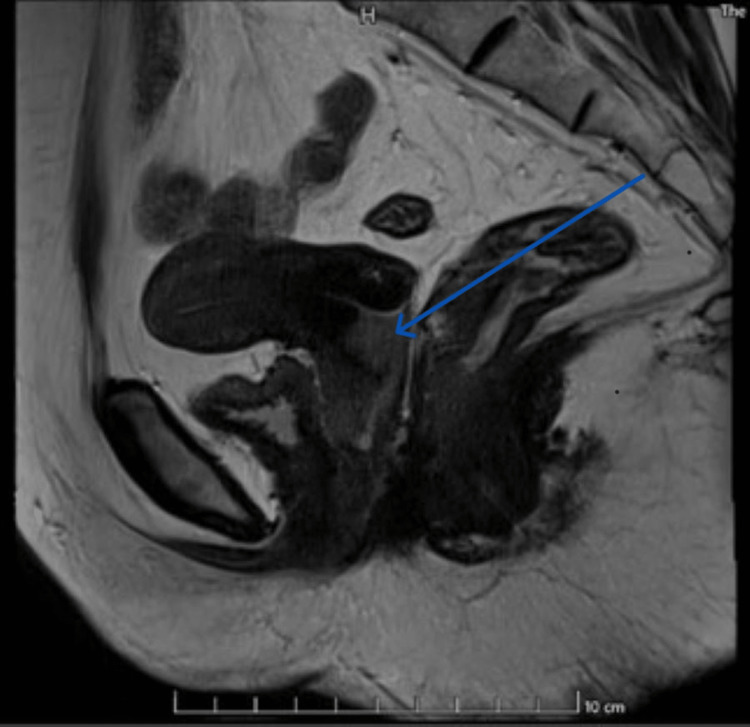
Magnetic Resonance Imaging-T2 Hyperintense Mass Lesion Cervical mass lesion showing no loss of fat plane between bladder and rectum.
